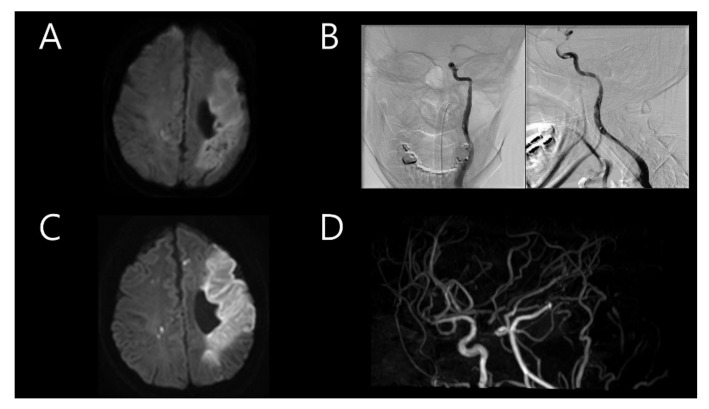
(A) Initial brain magnetic resonance imaging (MRI) after the first event showing stroke in the left middle cerebral artery (MCA) territory. (B) Immediate transfemoral cerebral angiography showing total occlusion of the distal portion of the left internal carotid artery (ICA). (C) Follow-up brain MRI on the following day showing acute infarction in the left MCA territory with multifocal embolic infarction in the bilateral hemispheres. (D) Magnetic resonance angiography showing complete occlusion of the left ICA.
